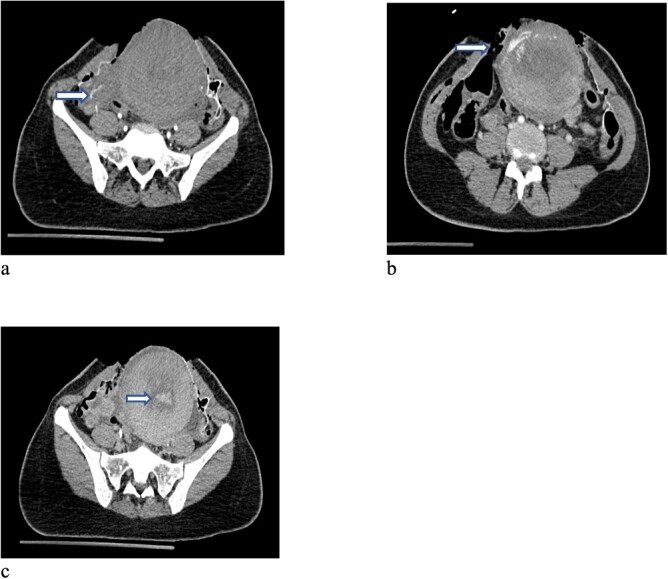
CT scan of the pelvis (a-c) after cesarean section revealed focus of contrast blush seen in the arterial phase at the right lateral side of the uterine wall, which increased in the venous and delayed phases suggesting active arterial bleeding. The contrast extravasation is also seen within the endometrial cavity.
